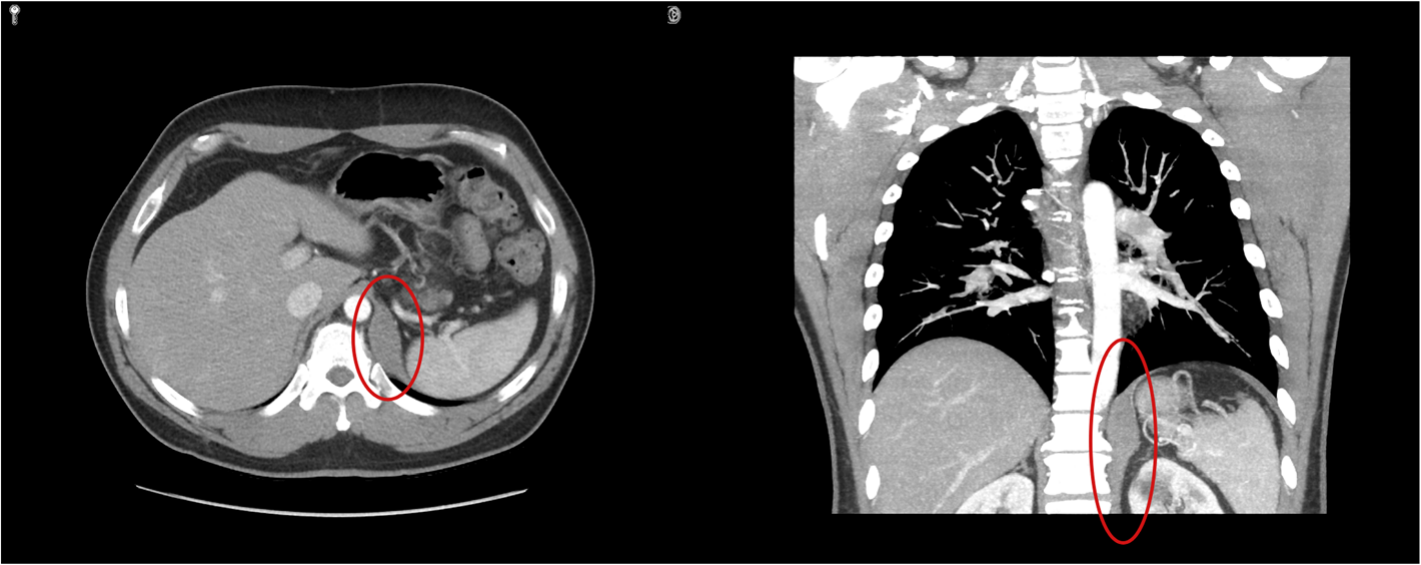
Axial and coronal slices of CT chest with contrast depicting a smoothly marginated and homogenous 5.3 cm × 2.3 cm × 5.9 cm soft tissue mass embedded within the muscle fibers of the crus of the left posterior medial hemidiaphragm with hounsfield of 60 units
